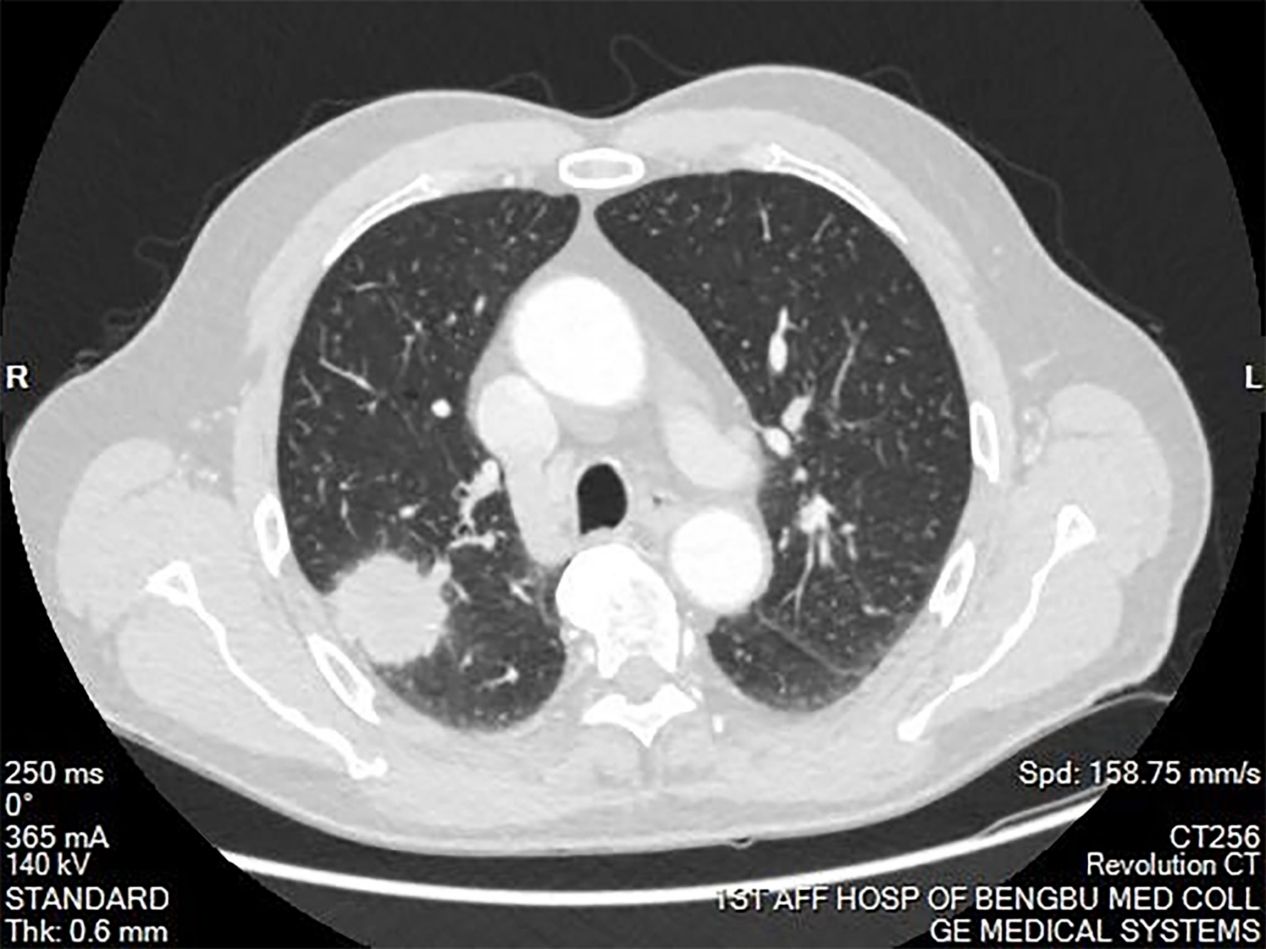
Computed tomography of the chest showing a mass in the right upper lobe of the lung. The mass was approximately 4cm in diameter. Burrs were present on the edge of the mass. A portion of the mass was connected to the pleura. The mass had uneven enhancement after the enhancement scan.
